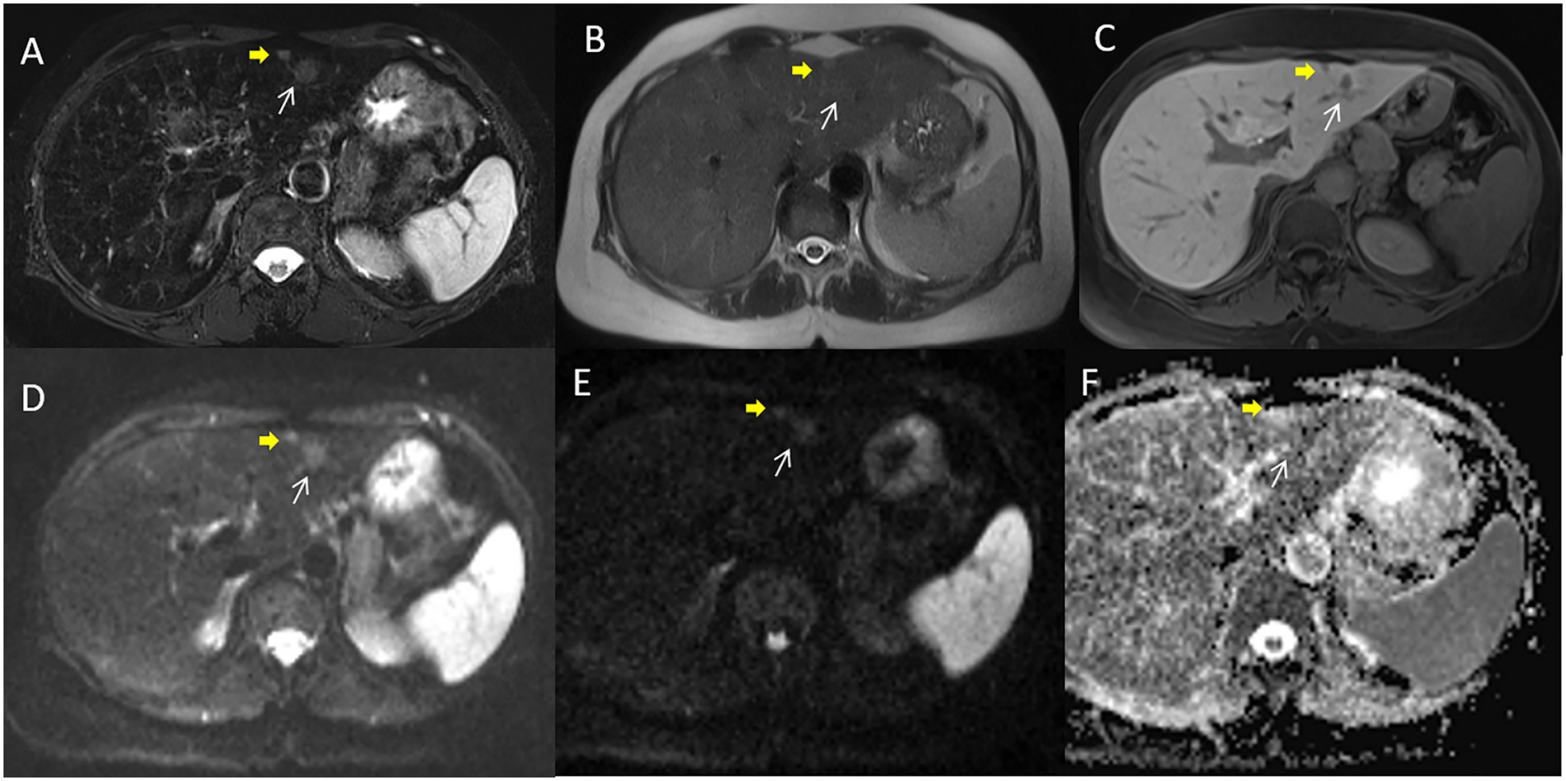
Woman 54 y with right colon cancer. Pre surgical MRI study: In A (SPACE FS T2-W sequence) white arrow shows parenchymal metastasis and yellow arrow shows subcapsular lesion. Parenchimal lesion is not detected by HAlf fourier Single- shot Turbo spin-Echo (HASTE) T2-W sequence (in B) while subcapsular lesion is detected. In EOB phase contrast study the metastases appear as hypointense lesions with restricted diffusion in DWI sequences and hypointense signal in ADC map (D, E and F).
